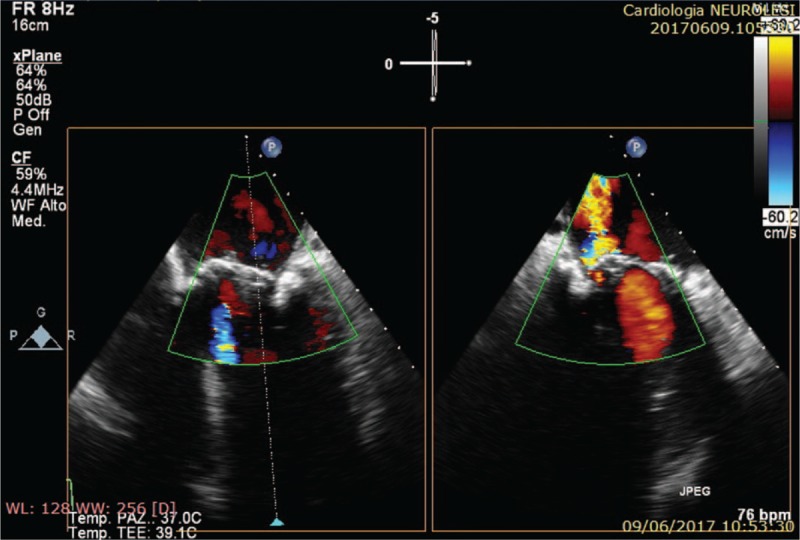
Transesophageal ecocardiography demonstrates a periprosthetic severe regurgitation.
